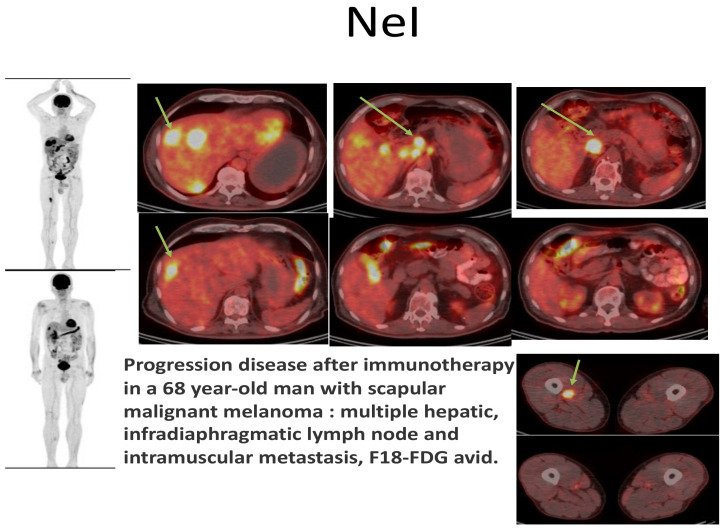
Malignant melanoma and various aspects of metastatic spread, as identified and depicted by PET-CT.
